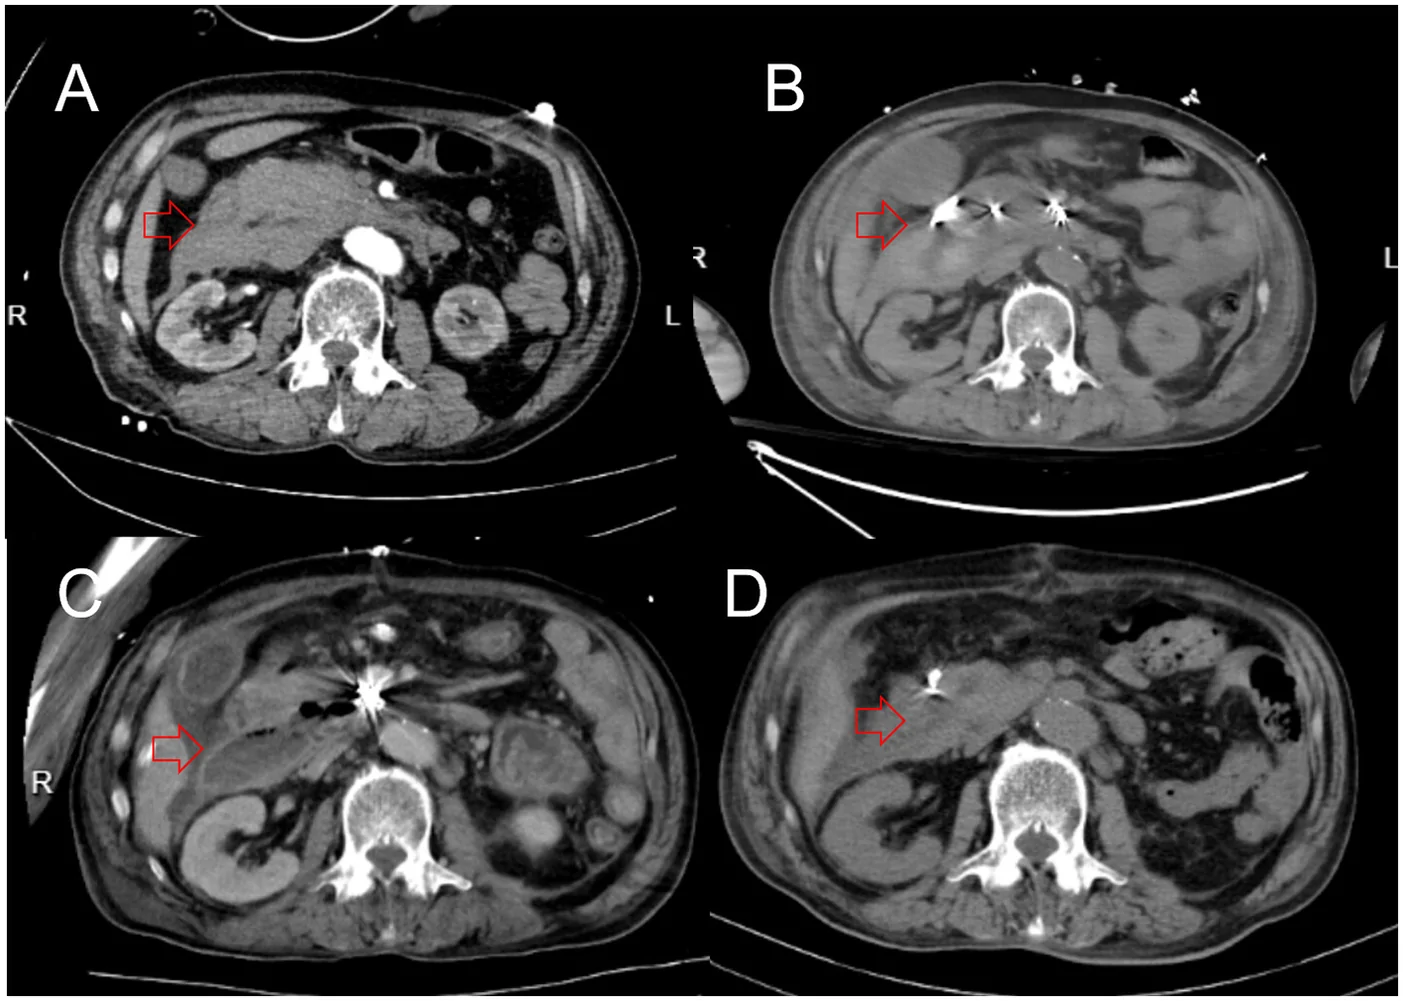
Serial abdominal CT findings. (A) Preoperative CTA showing a hyperdense area with nodular contrast extravasation (arrow) in the abdominal cavity, indicating active hemorrhage. (B) CT after interventional embolization and before exploratory laparotomy, demonstrating enlargement of the abdominal hematoma. (C) CT after exploratory laparotomy, showing evacuation of the hematoma and packing. (D) CT before discharge, revealing significant resolution of the hematoma.
